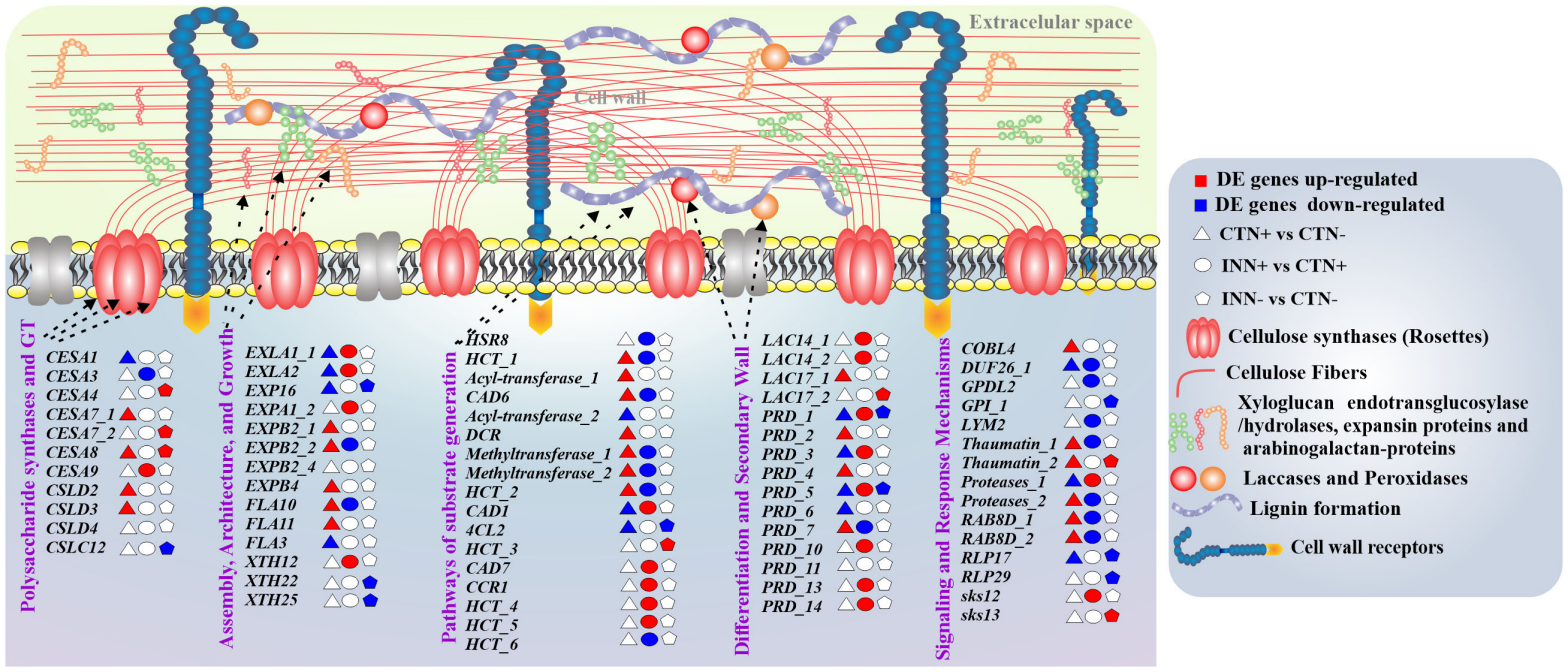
Overview of a scheme showing the regulation of cell wall components. This model presents the differentially expressed genes of maize plants inoculated with *H. seropedicae* ZAE94 (IN) or mock-inoculated (CT) and cultivated in 0.3 mM (N-) or 3 mM (N+) of N. Up-regulated (red colors) or down-regulated (blue colors). White Symbol represents expressed genes, but not differentially regulated. *CESA*, cellulose synthase, *EXLA*, expansin-like A; *EXP*, expansin; *EXPA*, expansin A; *EXPB*, expansin B; *FLA*, fasciclin-like arabinogalactan; *XTH*, xyloglucan endo-transglycosylase; *HSR8*, NAD(P)-binding Rossmann-fold superfamily protein; *HCT*, hydroxycinnamoyl-CoA shikimate/quinate hydroxycinnamoyl transferase; *CAD*, cinnamyl alcohol dehydrogenase; *DCR*, HXXXD-type acyl-transferase family protein; 4CL2, 4-coumarate-CoA ligase 2; *CCR1*, cinnamoyl-CoA reductase 1; *LAC*, laccase; *PRD*, peroxidase; *COBL4*, COBRA-like extracellular glycosyl-phosphatidyl inositol-anchored protein family; *DUF26*, Domain of unknown function; *GPDL2*, PLC-like phosphodiesterase family protein; *GPI*, Carbohydrate-binding X8 domain superfamily protein; *LYM2*, Lysm domain GPI-anchored protein 2 precursor; *RAB8D*, RAB GTPase homolog E1B; *RLP*, Leucine-rich repeat (LRR) family protein; *SKS*, SKU5 similar.
